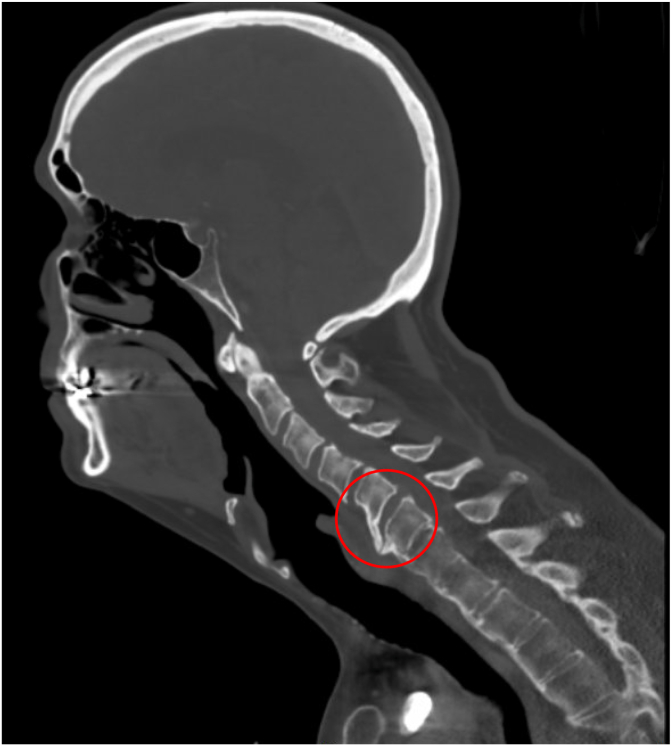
Sagittal stroke CT angiogram head and neck demonstrating a C5-6 osteophyte (red circle).
